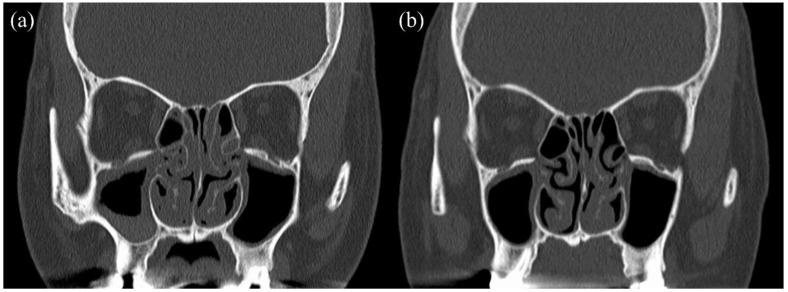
Sinus CT before and after sequential biotherapy: (a) before sequential biotherapy, (b) after sequential biotherapy for 7 months.
